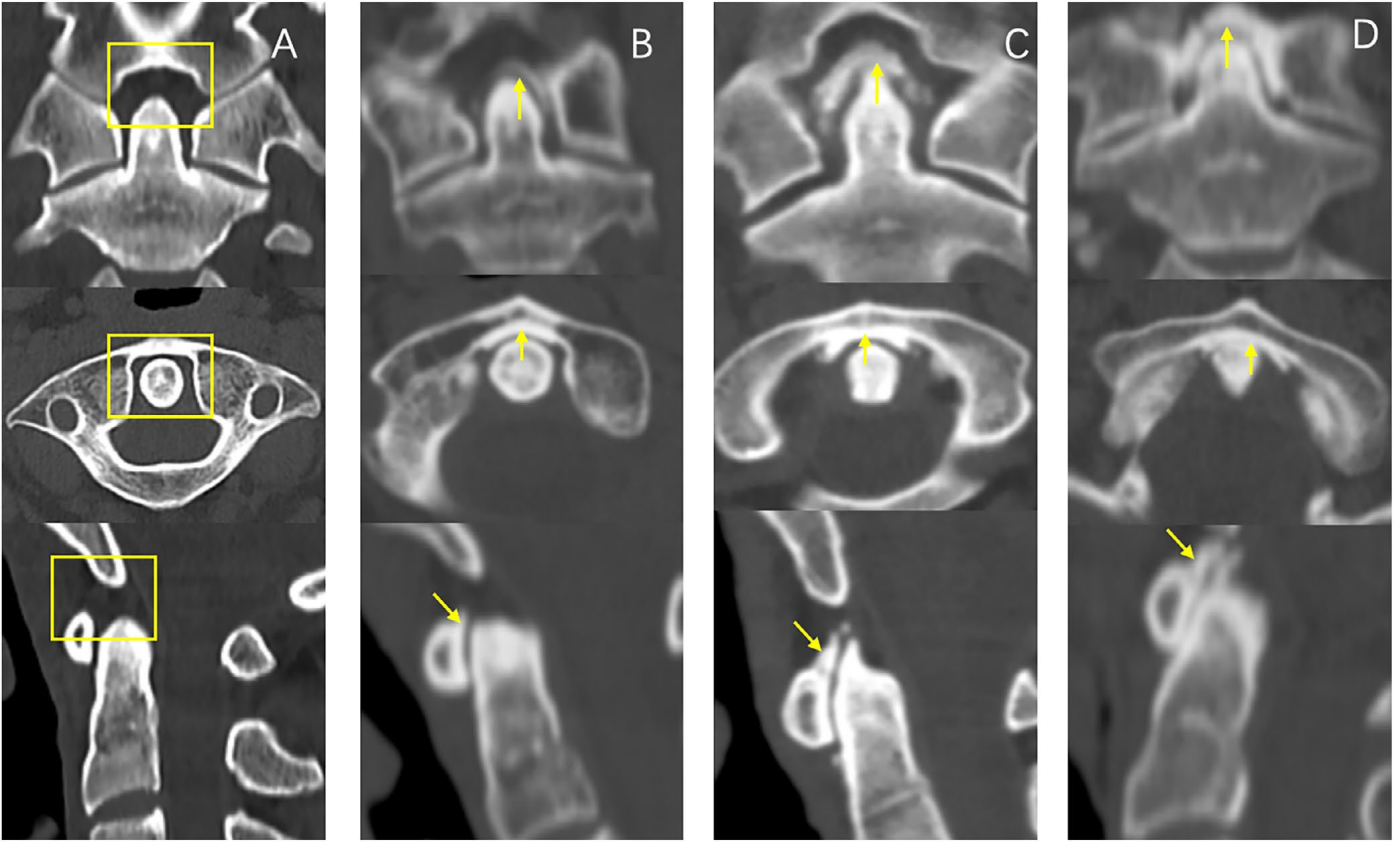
Evaluation of ossification of the atlanto‐occipital ligament. The different grades were based on the length of ossification of interest (XY) with respect to the distance from the posterosuperior rim of the anterior arch of the atlas to the inferior margin of the foramen magnum (AO) on mid‐sagittal cervical spine CT images. (A) Coronal, axial, and sagittal views from a patient with Grade 0 ossification of the atlanto‐occipital ligament. (B) Coronal, axial, and sagittal views from a patient with Grade 1 ossification of the atlanto‐occipital ligament. (C) Coronal, axial, and sagittal views from a patient with Grade 2 ossification of the atlanto‐occipital ligament. (D) Coronal, axial, and sagittal views from a patient with Grade 3 ossification of the atlanto‐occipital ligament.
